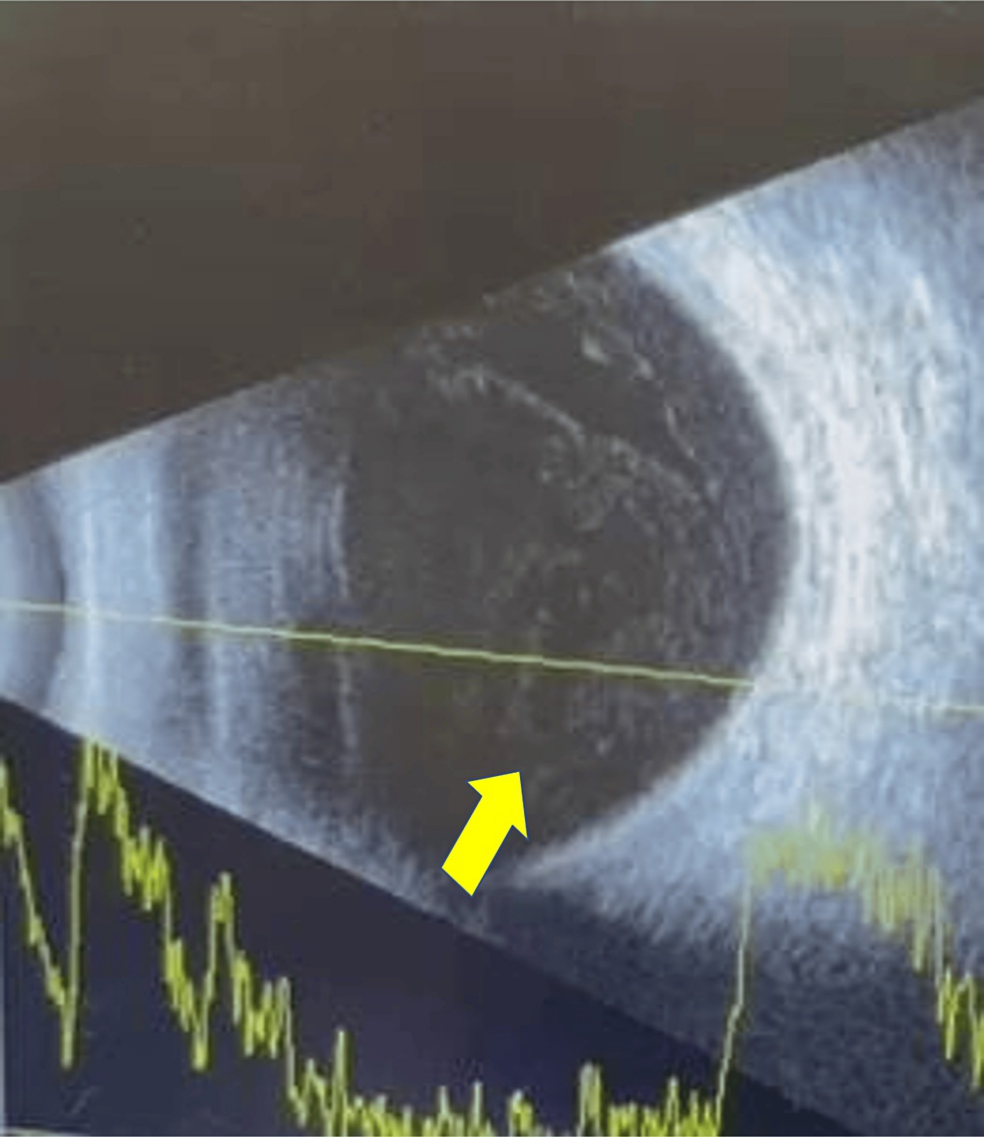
B-scan ultrasound of right eye with gain of 110 decibels showed heterogenous opacity with loculations (yellow arrow). The retina was flat.
